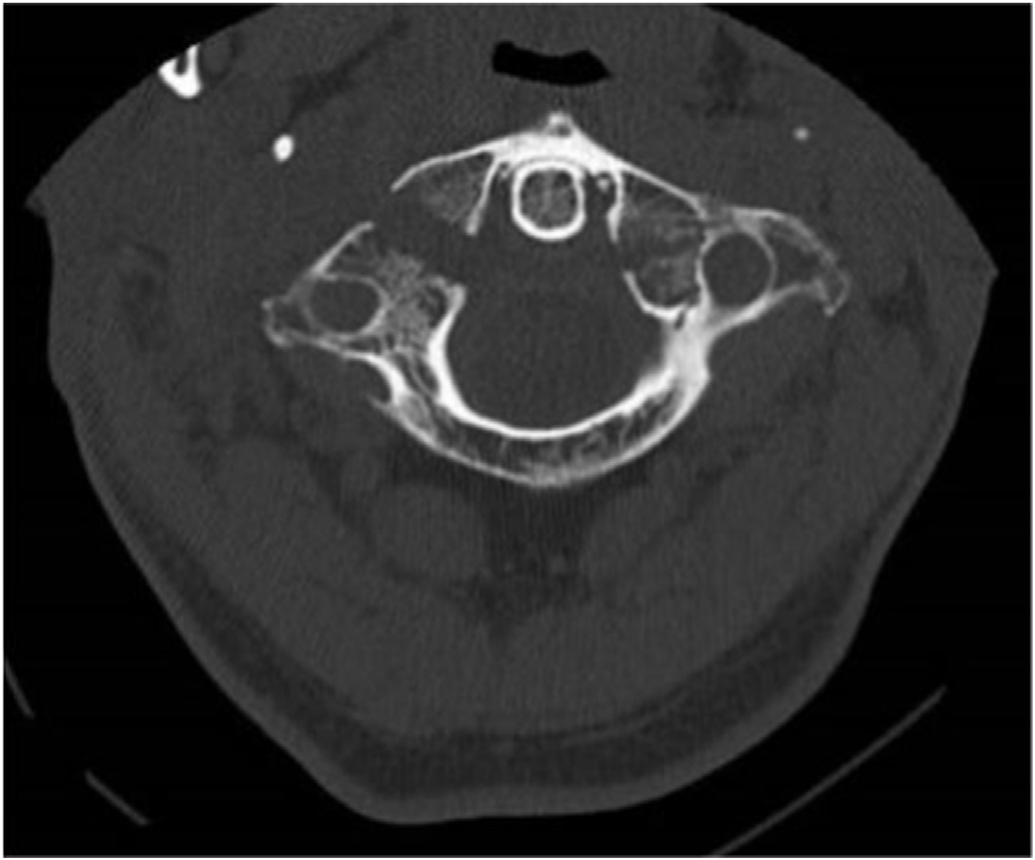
CT scan pre op: demonstrate severe bilateral fractures' edge gap.
